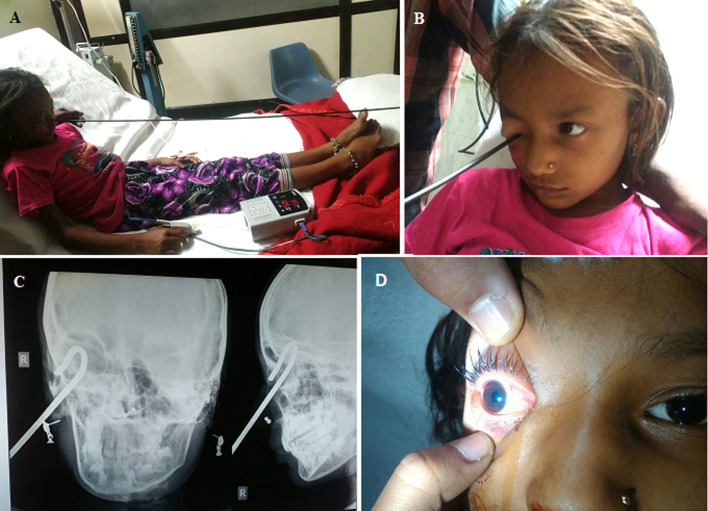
(A,B) traumatic eye injury with iron rod intact; C) X-ray showing iron rod blunt end being hooked inside the right orbit; D) post iron rod removal
